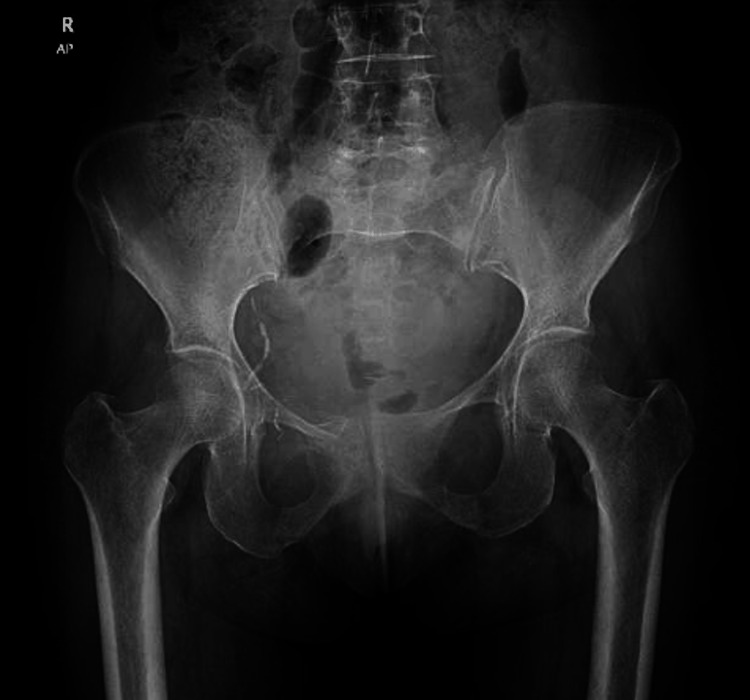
Pelvic X-ray displaying the initial fractures in the healing process with a well-healed left IPR fracture with callus formation and a misaligned right SPR. Additionally, linear density can be seen projected on the right side of the pelvis, likely postembolization IPR: inferior pubic rami; SPR: superior pubic ramus
